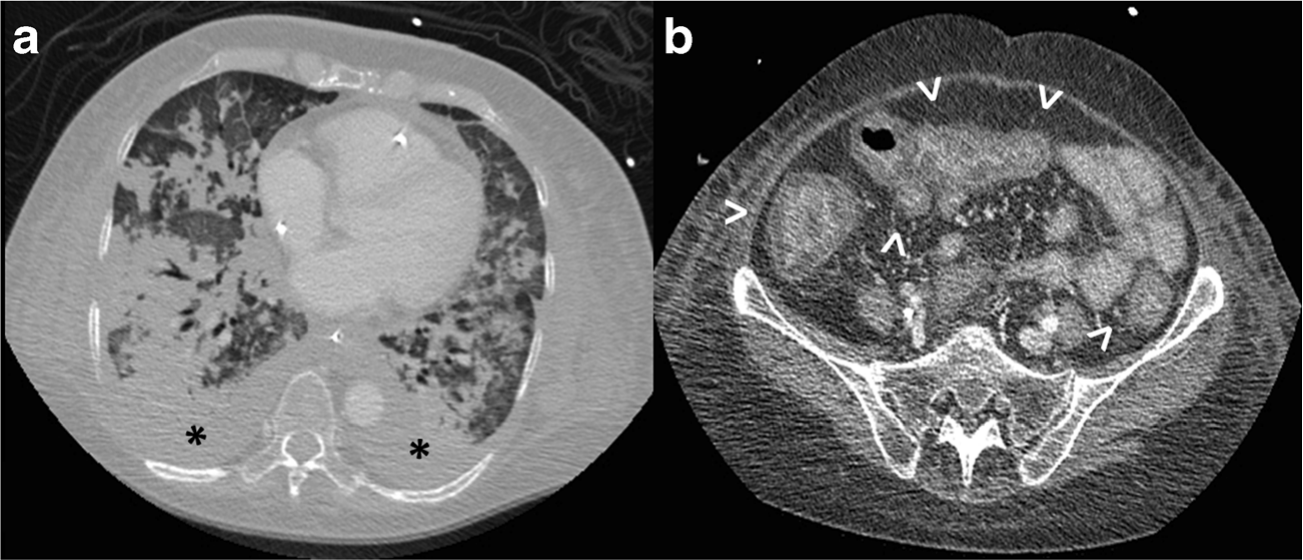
Strongyloidiasis. a. Chest CT
